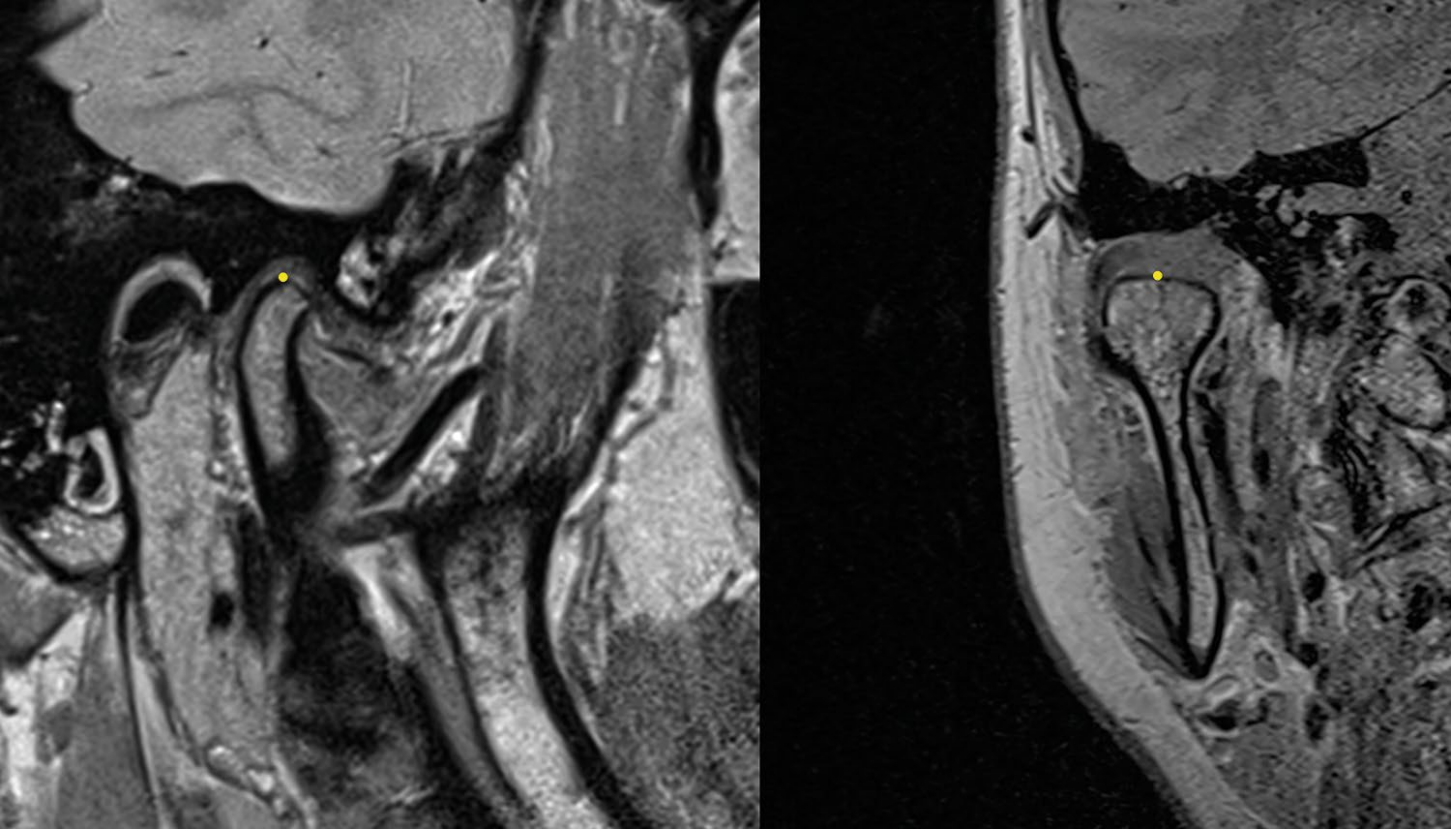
MRI scans in the parasagittal (left) and paracoronal views (right). Yellow dots indicate measuring points on central condyle slices.
